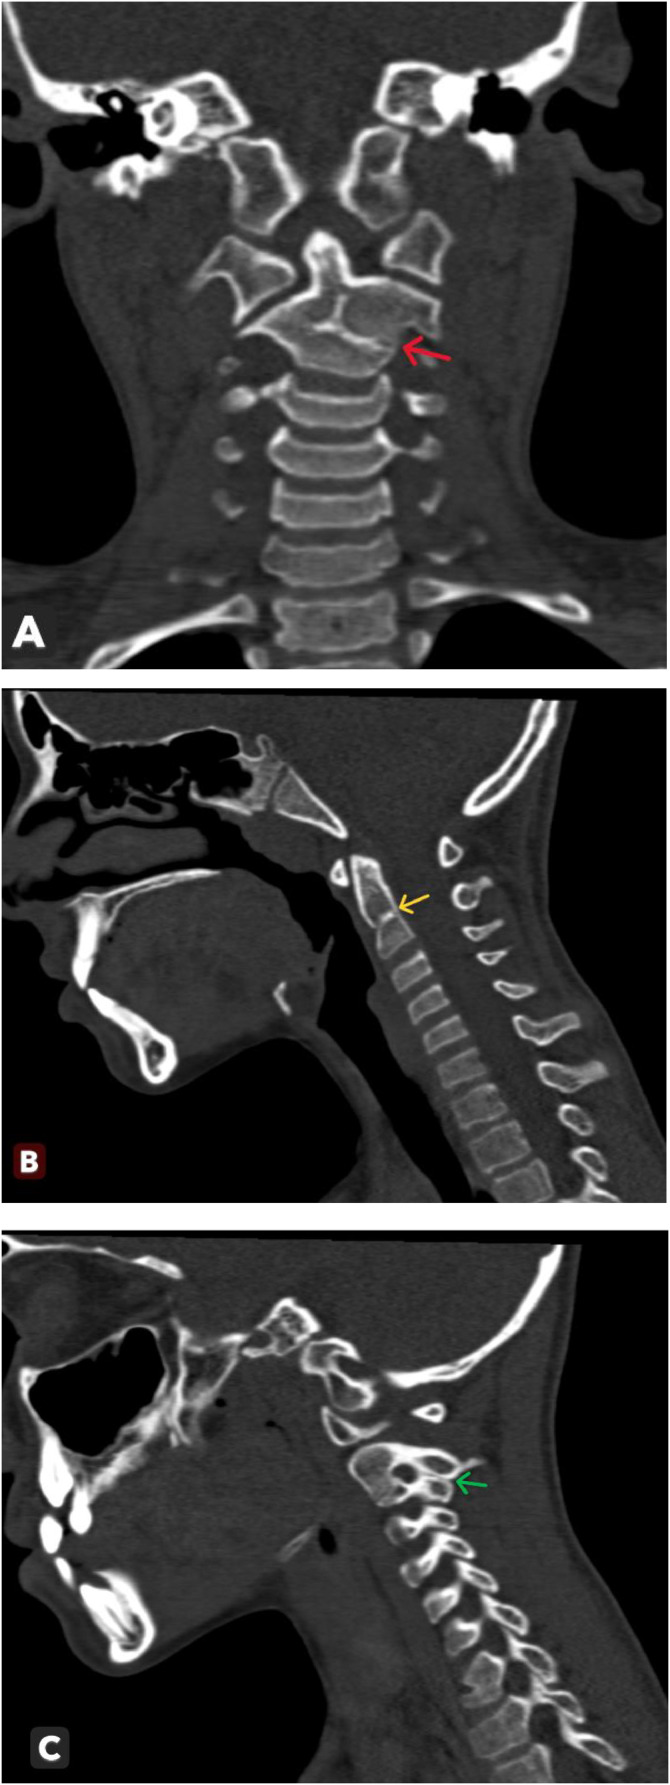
Coronal (A) and sagittal (B and C) computed tomography images of the cervical spine showing straightening of the cervical spine with fusion (block) of the C2-C3 vertebrae (red and yellow arrows), associated with two left transverse processes (green arrow).
